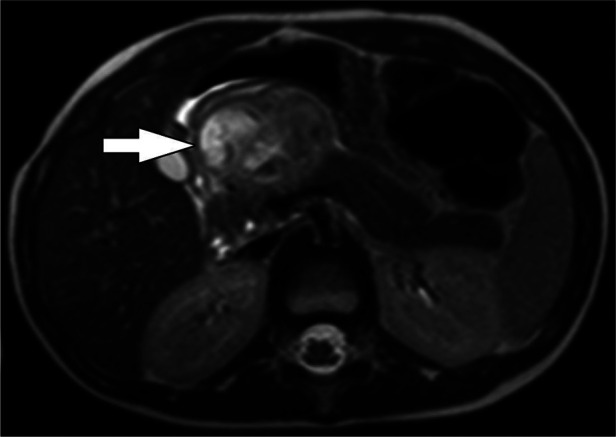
Pancreatic NET. Axial T2-weighted MRI shows a heterogeneously hyperintense exophytic mass arising from the neck of the pancreas (arrow). The upstream pancreatic body and tail are Normal and there is no duct dilation to suggest duct obstruction.
